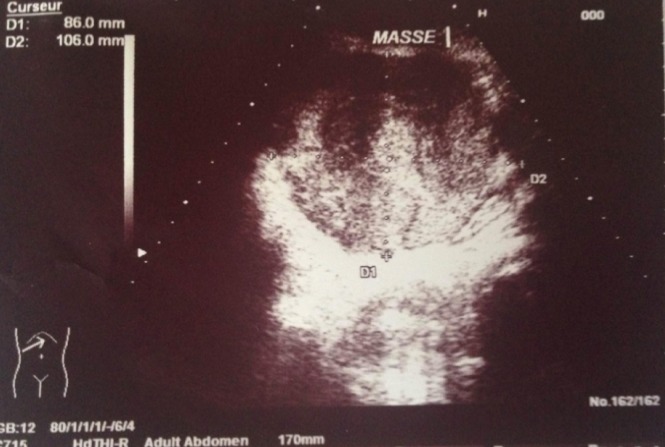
Abdominal ultrasonography: heterogeneous masse probably depending of the right hepatic lobe
